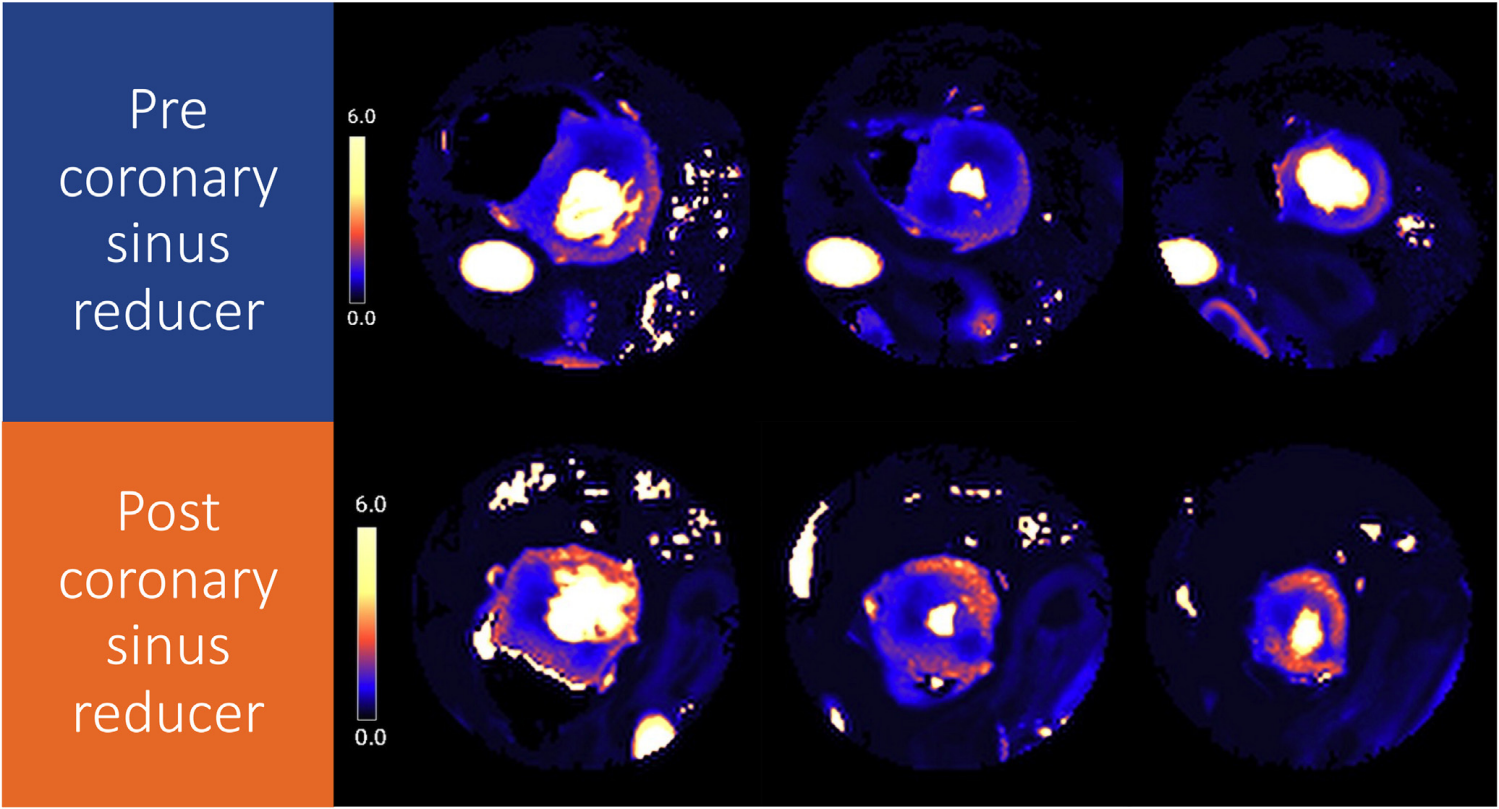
Cardiac magnetic resonance imaging pixel-wise quantitative stress perfusion maps of the basal, mid-, and apical ventricle before and after coronary sinus reducer. On the preprocedural images there is severe global hypoperfusion, most pronounced in the areas of maximal hypertrophy in the basal and mid-anteroseptum and inferior segments. After the procedure there is a significant increase in the global blood flow. Although there is persistent hypoperfusion in the anteroseptum and inferior wall, the extent and severity of this has reduced.
